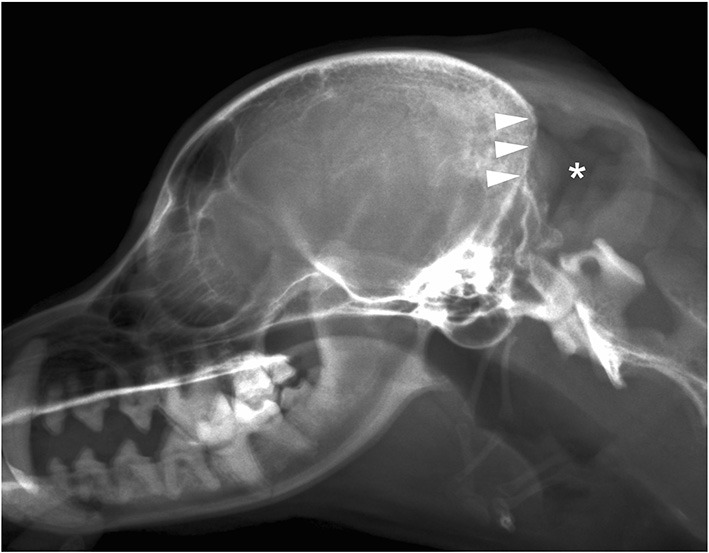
Plain X-ray film of the lateral view of the head. Radiolucent mass shadows in the occipital region (*) and opacification of the occipital bone (arrowhead) were observed.
